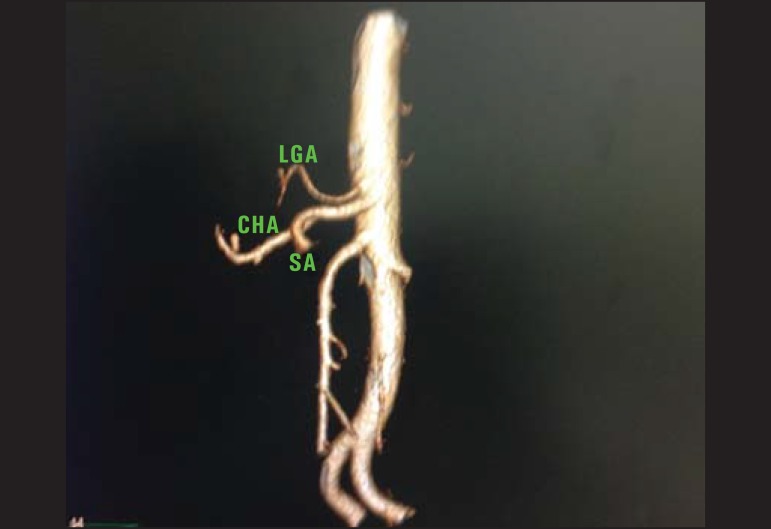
Contrast-enhanced CT with volume rendering demonstrating hepatosplenic
